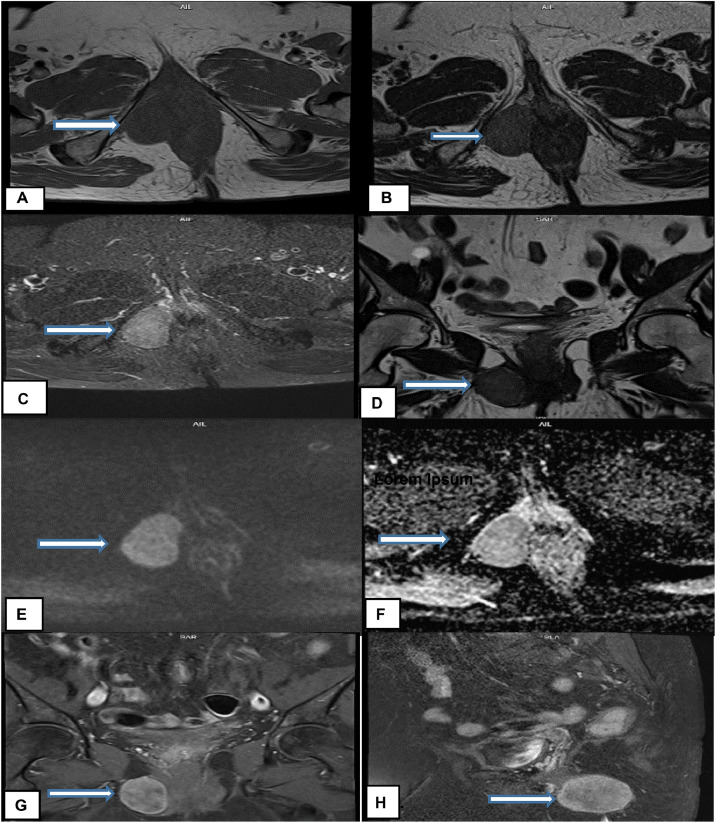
Magnetic resonance imaging of the ischioanal mass (white arrow). (A) Axial T1 weighted. (B) Axial T2 weighted. (C) Axial short tau inversion recovery (STIR). (D) Coronal T2 weighted. (E) Axial diffusion-weighted imaging (DWI). (F) Axial apparent diffusion coefficient (ADC) mapping. (G) and (H) postcontrast axial and sagittal imaging. A well-circumscribed iso to high T1W and intermediate T2W in the ischioanal space, which seemed to be intramuscular without invasion to adjacent structures such as the rectum.
